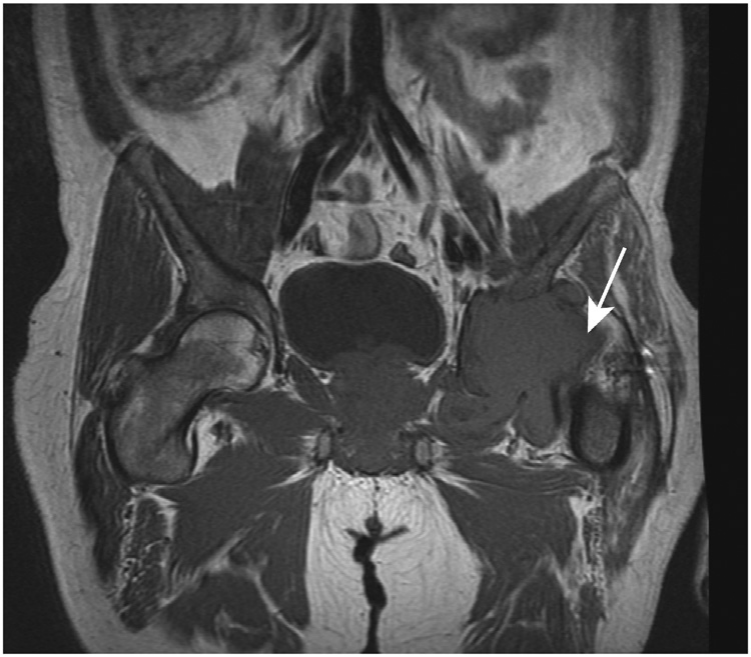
Magnetic resonance imaging of the pelvis.
